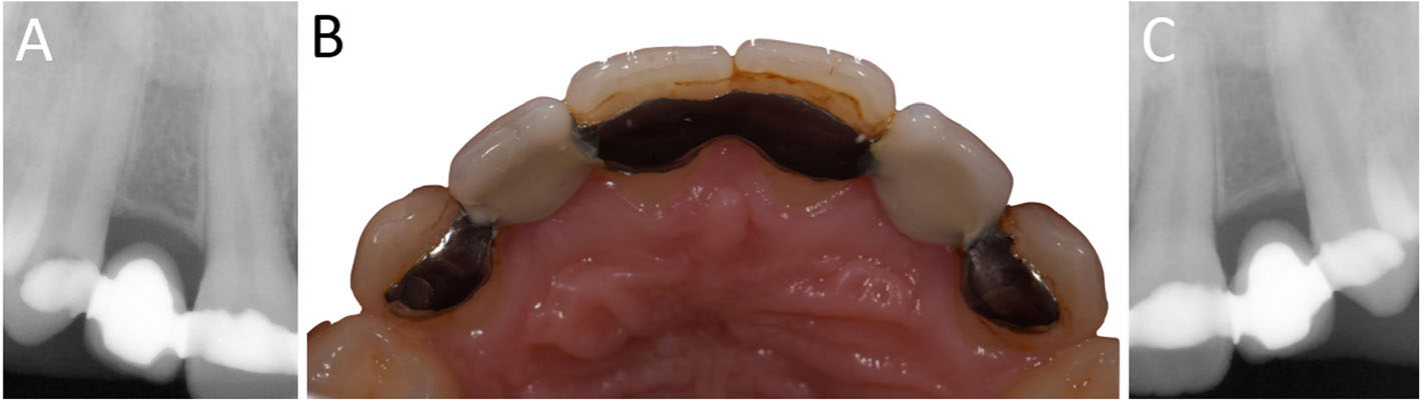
Pre-operative situation. The young patient presented with an old Maryland bridge, and asked the surgeon to replace it with two fixed implant-supported restorations. a Right side, radiographic control. The horizontal space between the roots of the adjacent teeth was narrow. b The Maryland bridge in position, occlusal view.; c Left side, radiographic control. The horizontal space between the roots of the adjacent teeth was narrow
